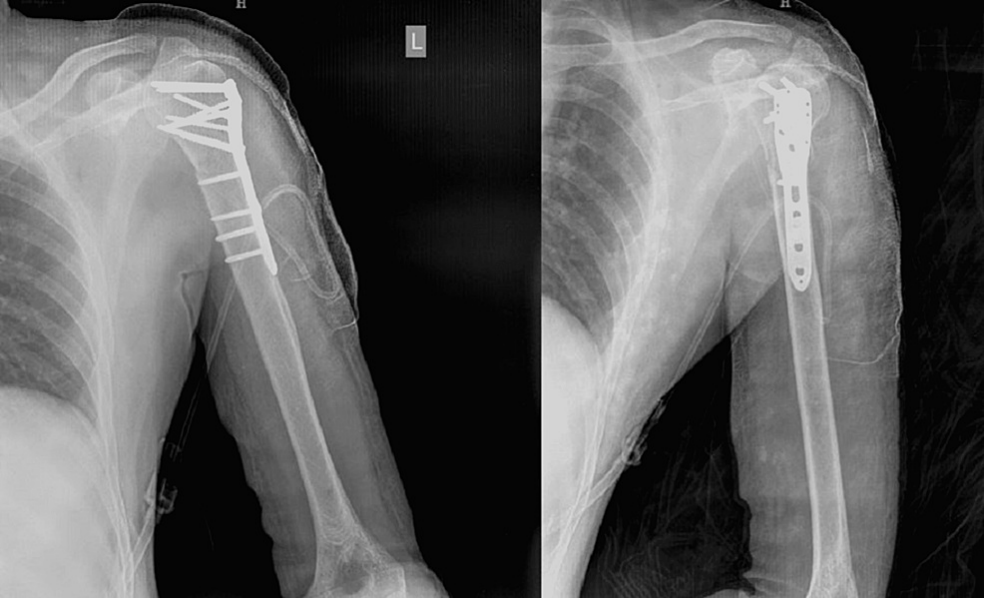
Postoperative X-rays of PHILOS fixation for proximal humerus fracture through the deltopectoral approach PHILOS: Proximal Humeral Interlocking System
